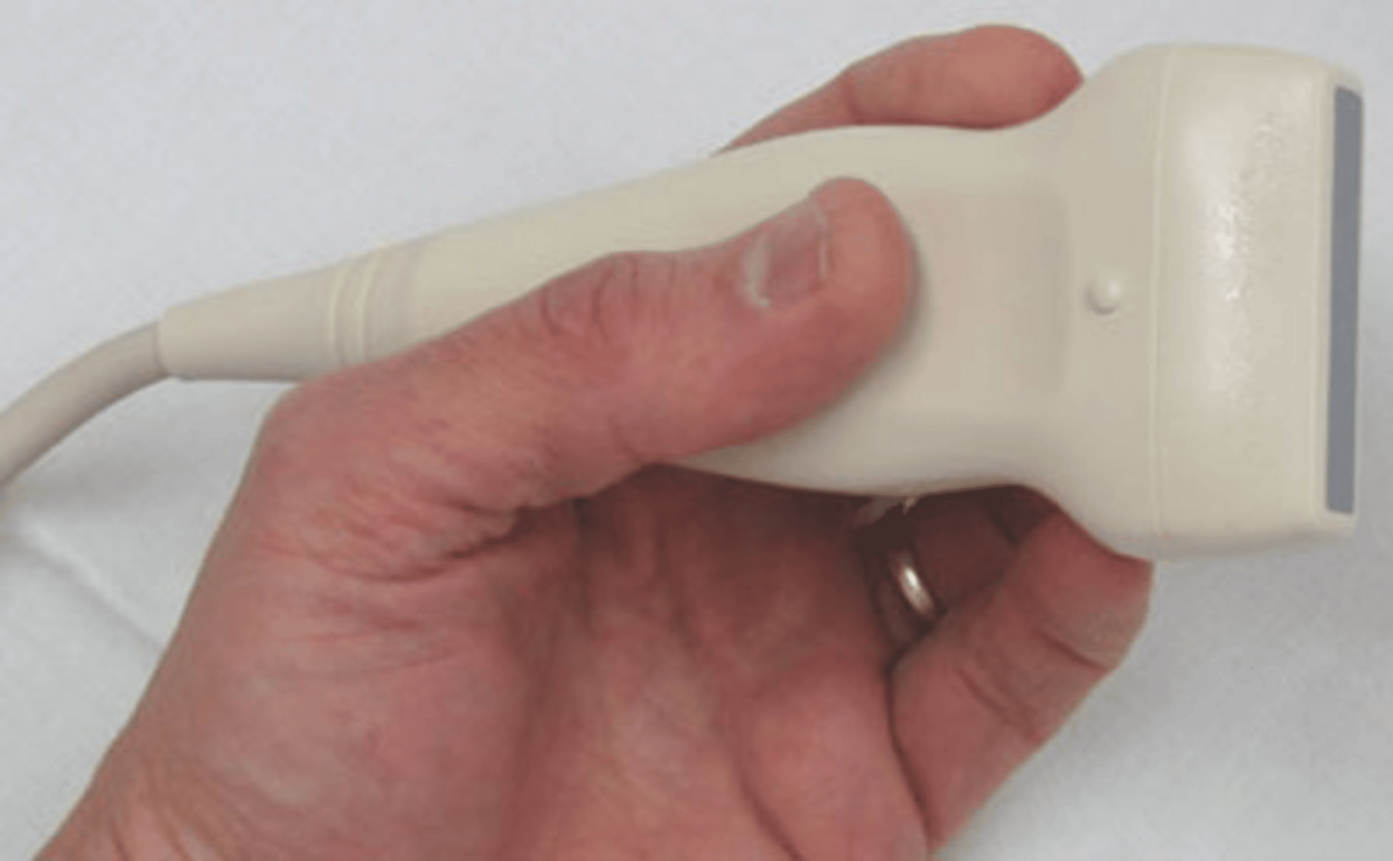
Linear ultrasound probe Image adapted from: Moradmand H. Ultrasound scanner and probe. Available from: https://openverse.org/image/48d9722e-08cf-4492-9875-d7d86e9ba074?q=ultrasound+probe&p=3. Licensed under CC BY-SA 2.0
